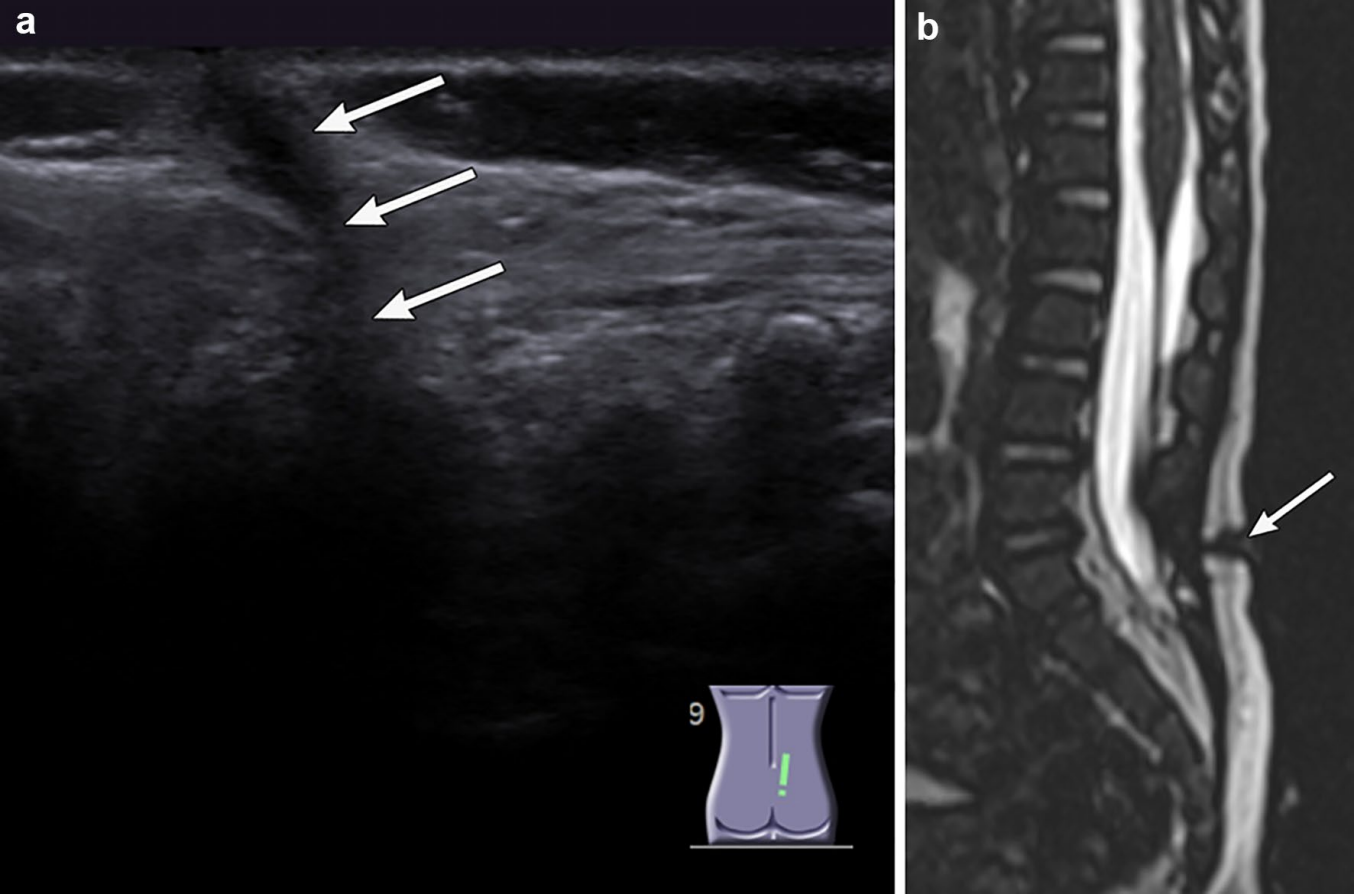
A spinal dermal sinus tract (DST) in a 1-year-old boy with bacterial meningitis (BM). A sacral dimple with cutaneous stigmata was found at 1 month. Initial spinal ultrasound (US) performed for screening purposes at 1 month did not detect any obvious abnormalities. The patient was admitted due to BM, and spinal US was performed again when the patient was 1 year old. a US at age 1 year shows a continuous tract to the spinal canal from the skin (white arrows). b Sagittal T2-weighted magnetic resonance image clearly shows a DST connected to a skin dimple from the dural sac (white arrow)
